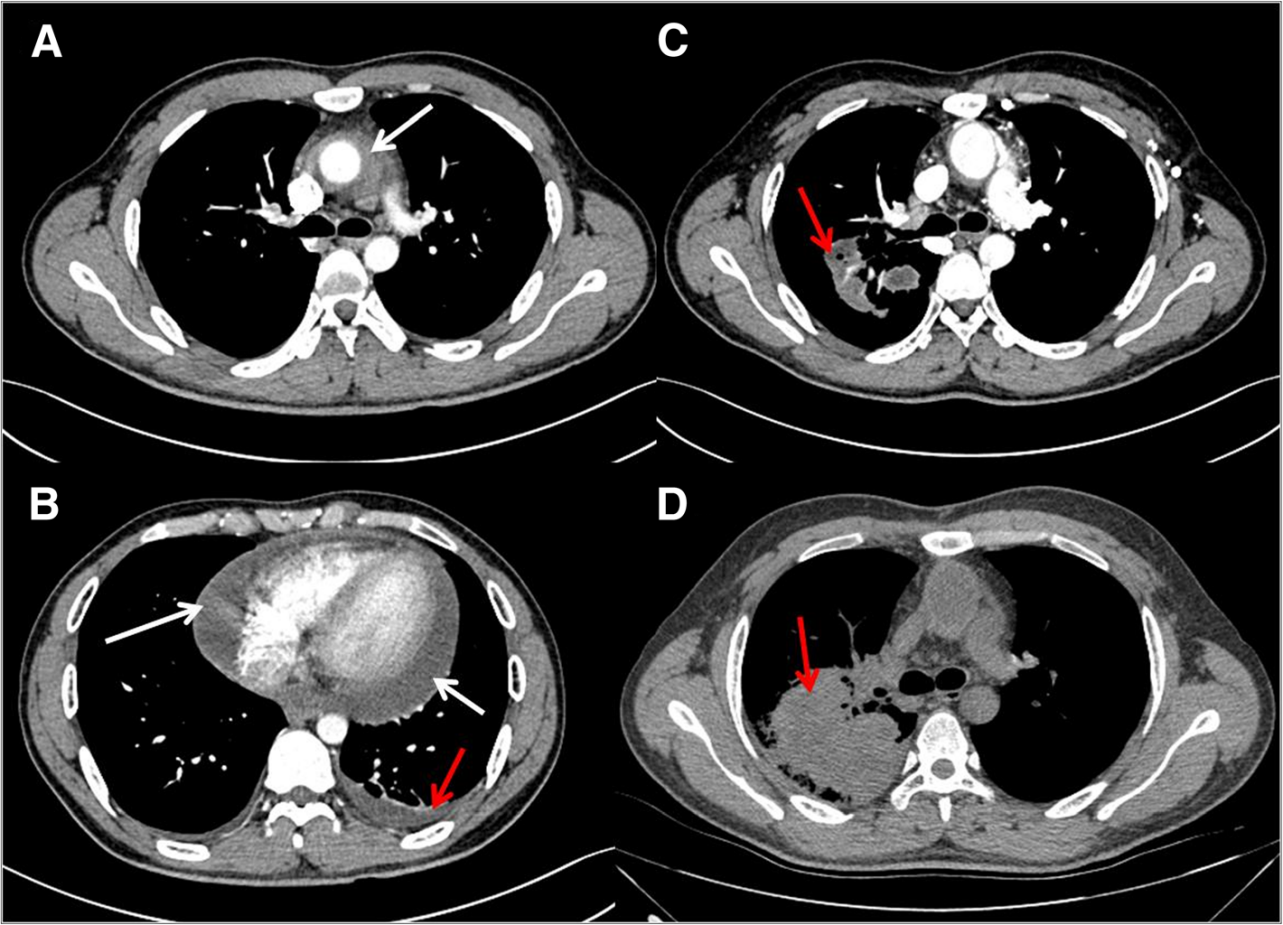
Computed tomography scan of the aorta shows a thickened aortic wall, pulmonary lesions, bilateral pleural effusion, and pericardial effusion: a Ascending aortic dissection and thickened aortic wall (white arrow); b Bilateral pleural effusion (red arrow) and pericardial effusion (white arrow); c pulmonary lesions (red arrow); d pulmonary lesions (red arrow)
